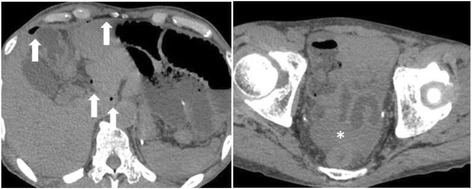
Free air and fluid in the peritoneal cavity visible on abdominal CT
